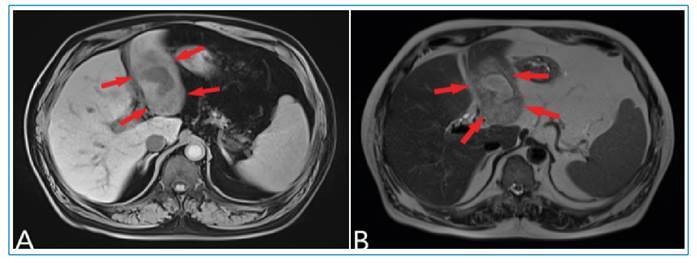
(A) Hypointense lesion detected on T1-weighted images extending inferiorly at the second segment of the liver (arrows); (B) Hyperintense lesion on T2-weighted images (arrows).
